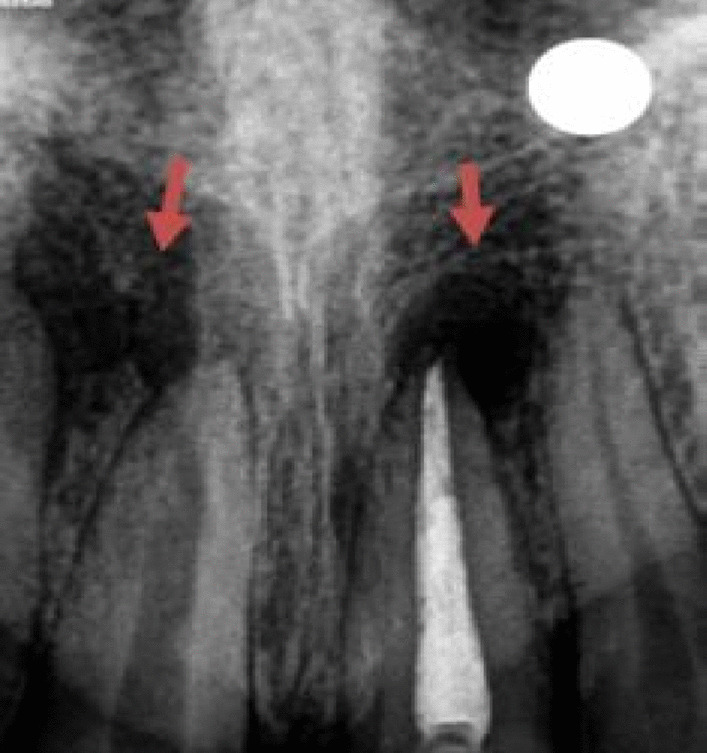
Preoperative periapical radiograph showing 4 to 5 mm of radiolucency around the previously root-treated tooth 21 and 11 with an open apex
